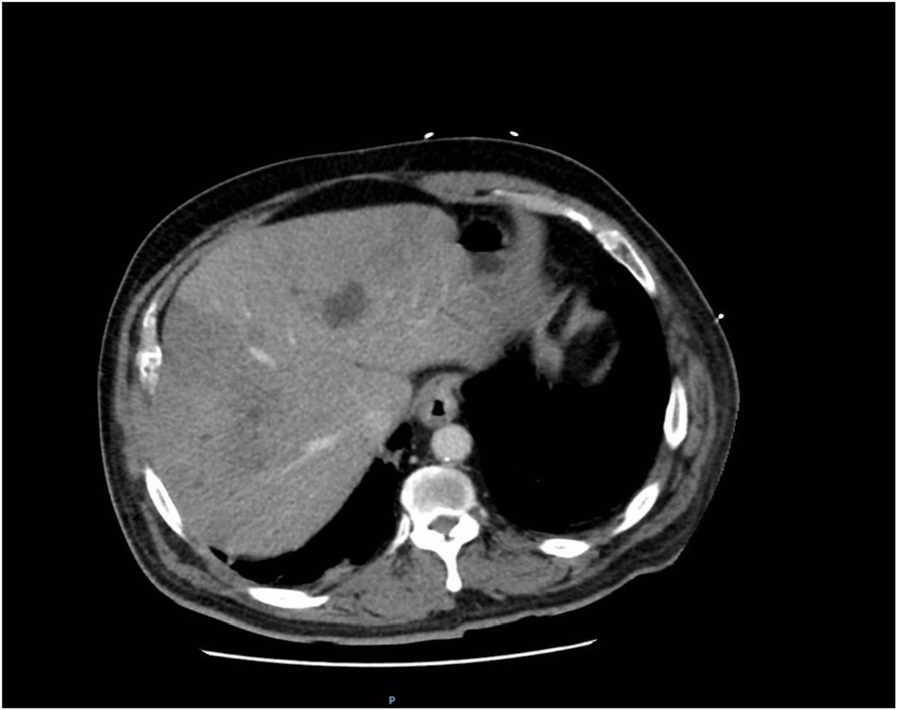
Computed tomography (CT) of the abdomen and pelvis 72 hours after presentation to the hospital showing multiple lobulated intrahepatic collections, infarction of hepatic segments 5 and 8, and septic occlusion of the portal vein. There were no gas locules associated with the abscesses. CT also demonstrated small bowel enteritis (not shown)
